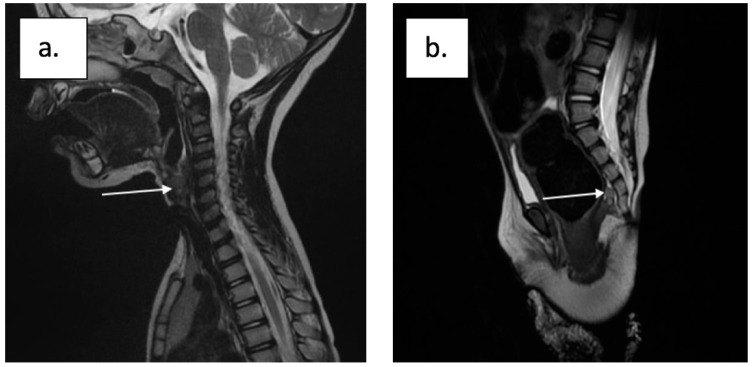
Magnetic resonance image showing (a) airway obstruction, (b) coccyx agenesis, and presacral teratoma.
